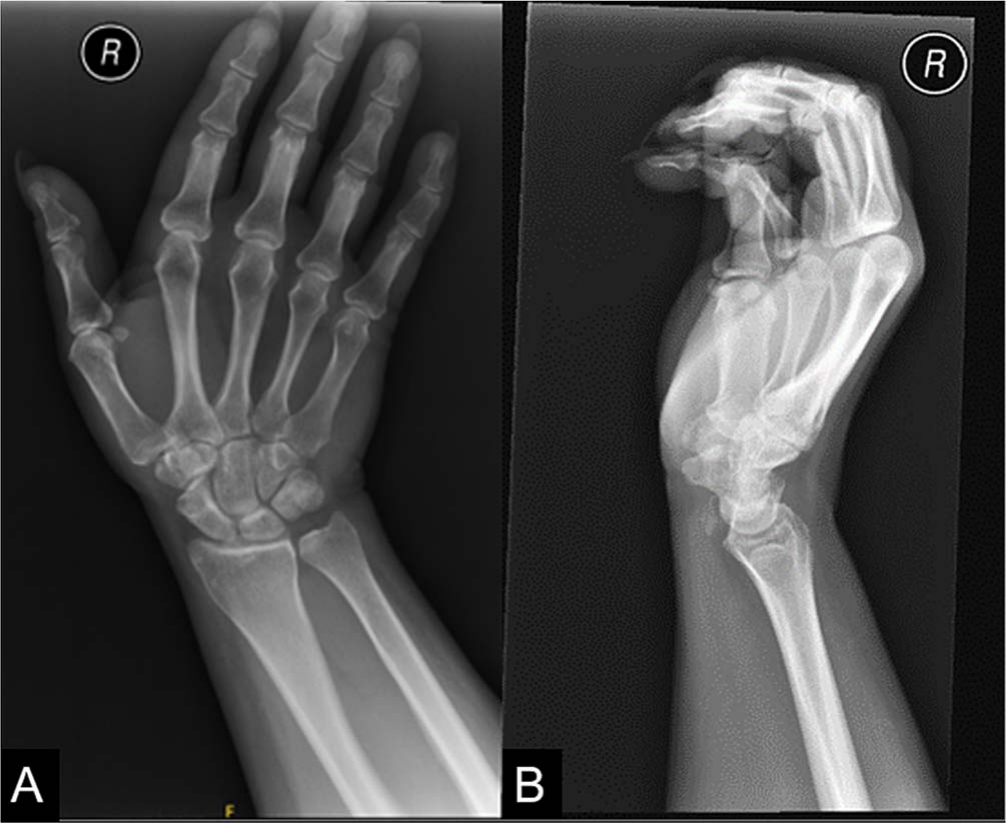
An X-ray of the right wrist which shows calcification at the level of flexor carpi radialis at the wrist.
